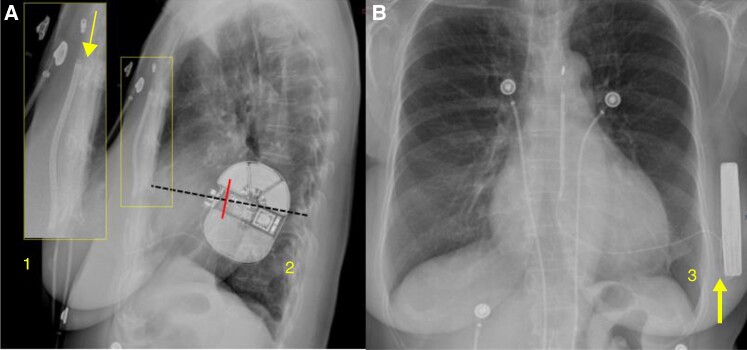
Chest radiograph analysis according to the PRAETORIAN score. In *A*, the thickness of the adipose tissue is ≤1 coil width. This corresponds to 30 points in the score system (Step 1 of the PRAETORIAN score) and low predicted risk of shock failure. The lateral view also allows to evaluate the generator placement in relation to the midline (Step 2 of the PRAETORIAN score). The S-ICD generator is in an optimal position, posterior to the midline; the score is, therefore, multiplied by 1. In *B*, the postero-anterior chest radiograph view allows to determine the amount of sub-generator fat (Step 3 of the PRATEORIAN score). As a reference, the generator width is used. In the example, <1 generator width of fat tissue is observed between the nearest point of the generator and the thoracic wall. The score is multiplied by 1.
